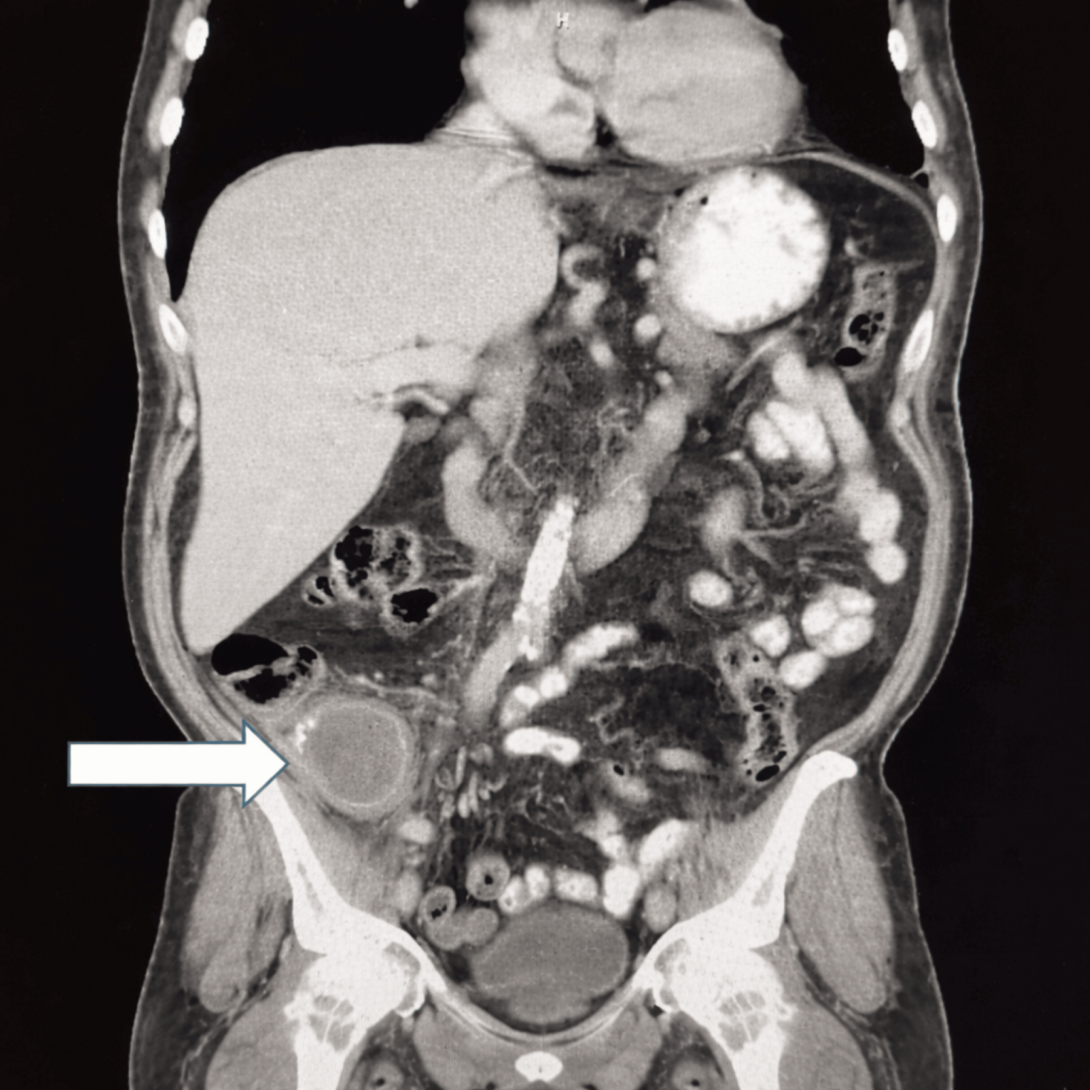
CT of the abdomen and pelvis with contrast showing appendiceal perforation and a rim-enhancing abscess in the right lower quadrant (arrow)
